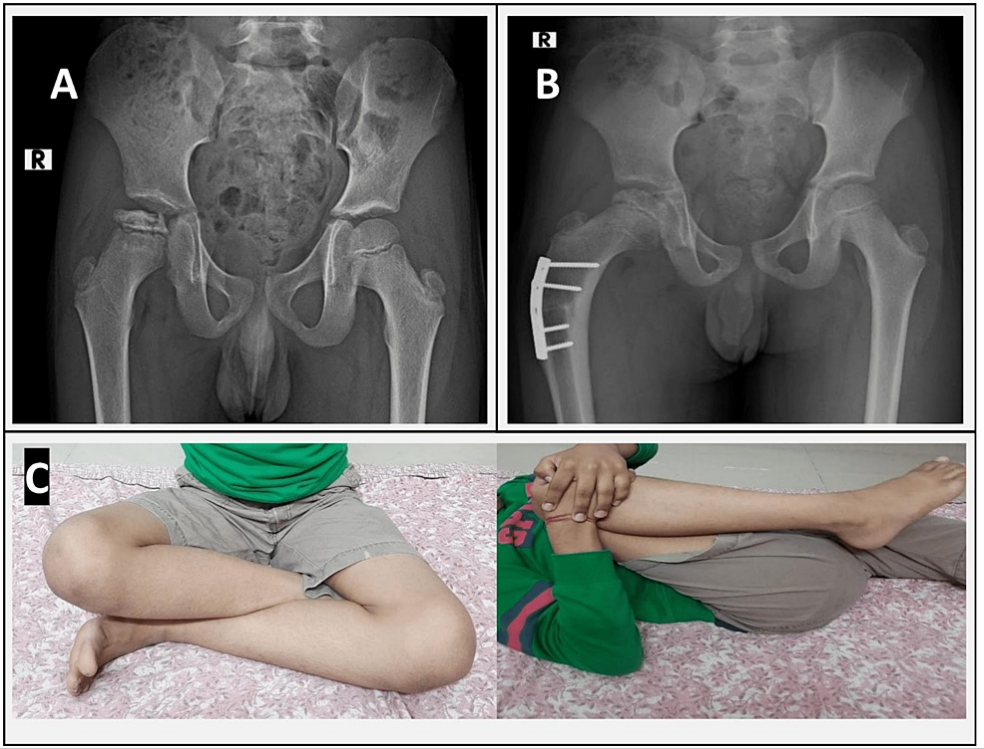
An eight-year-old boy with LCPD. (A) Preoperative radiograph showing modified Elizabethtown Stage IIB. (B) Postoperative radiograph at a follow-up of two years. (C) Range of movement found satisfactory at a follow-up of two years. LCPD: Legg-Calve-Perthes Disease.
